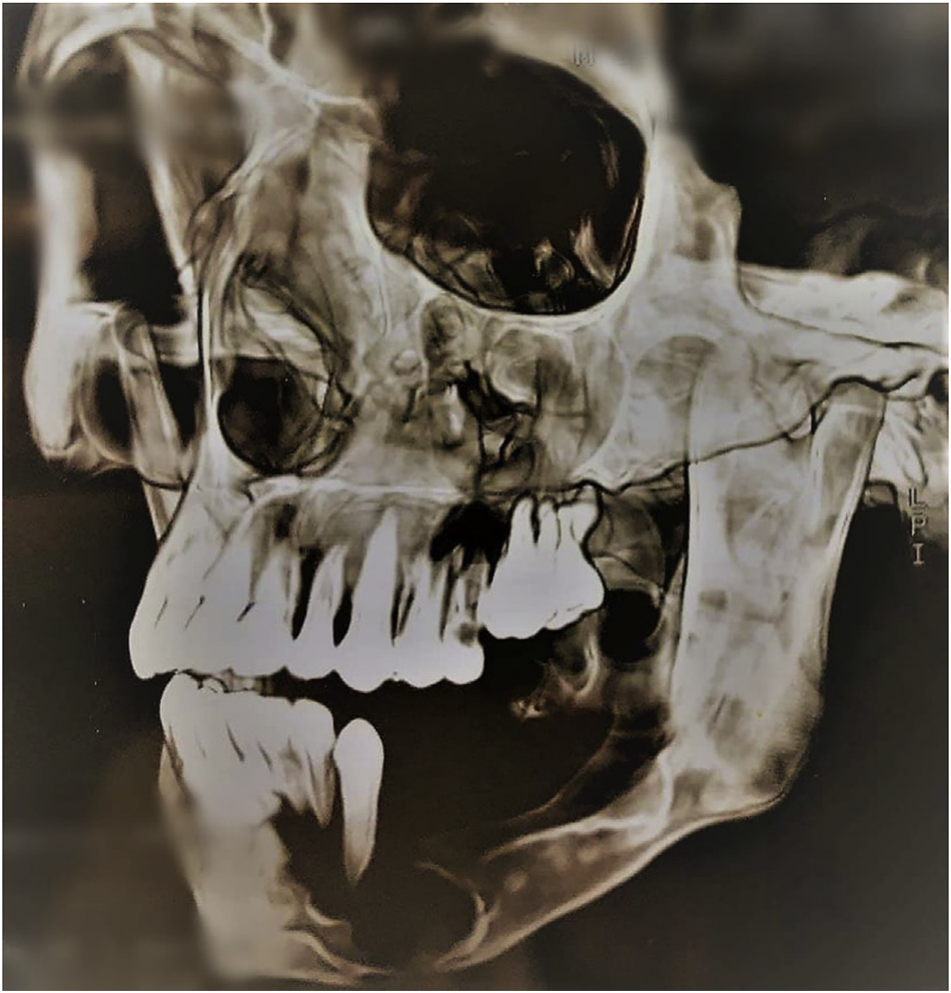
CT scan with 3D reconstruction :3/4 incidence showing multiple radiolucent areas with well outlined borders on the mandible with a fracture and on the maxilla.
